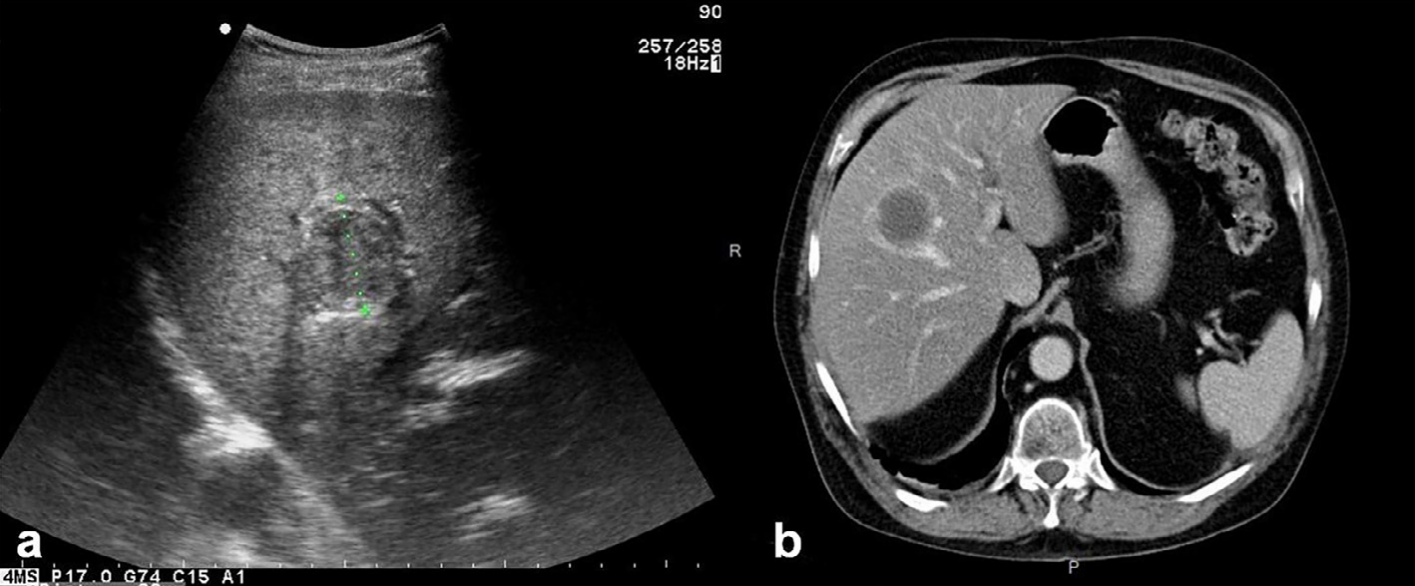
Alveolar echinococcosis in a patient with immunosuppression.* (a) Abdominal gray-scale US shows an abscess-like hepatic image. (b) Axial unenhanced CT shows a typical aspect of pyogenic liver abscess.
